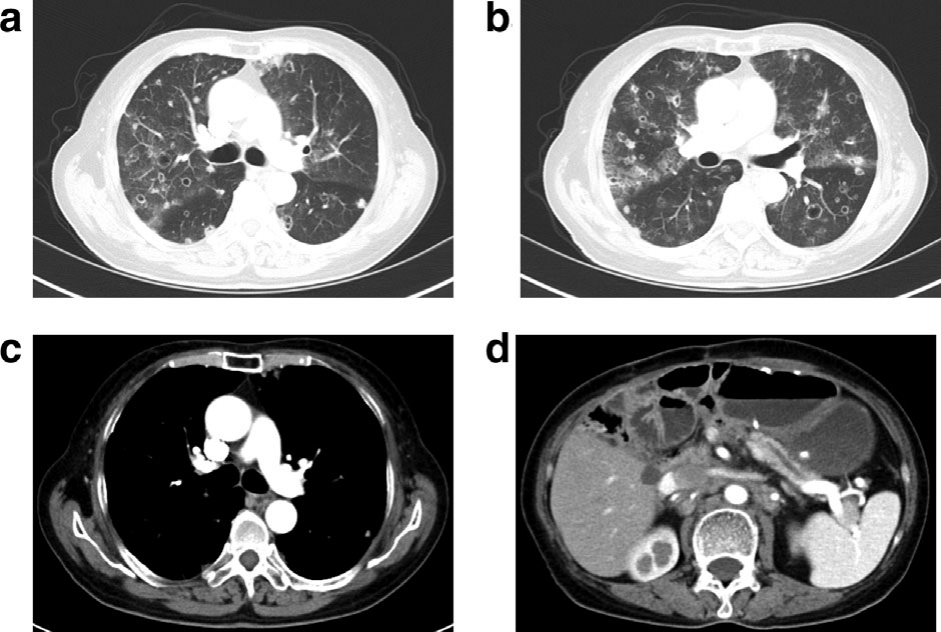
Enhanced CT image of chest and abdomen on 15 April 2018. (a, b) multiple cavitary shadows of bilateral lungs (arrow); (c) No enlarged lymph nodes were found under the carina or in the mediastinum; (d) Disordered structure of the pancreatic head area, dilated pancreatic duct, and gas accumulated in some intrahepatic bile ducts.
